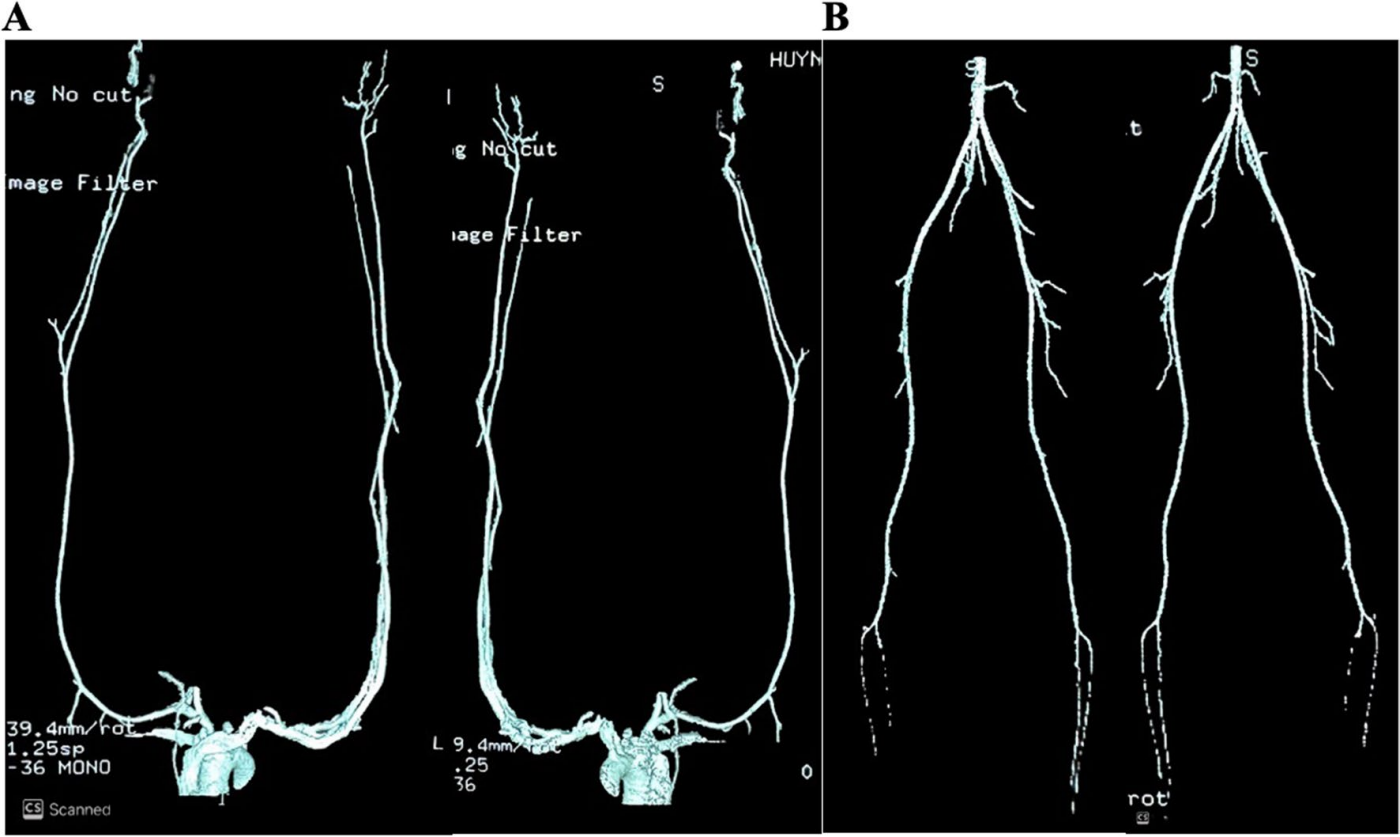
Extremity angiography on computed tomography. A Normal angiography of the upper limbs. B Occlusion of the anterior and posterior tibialis arteries without evidence of atherosclerosis
